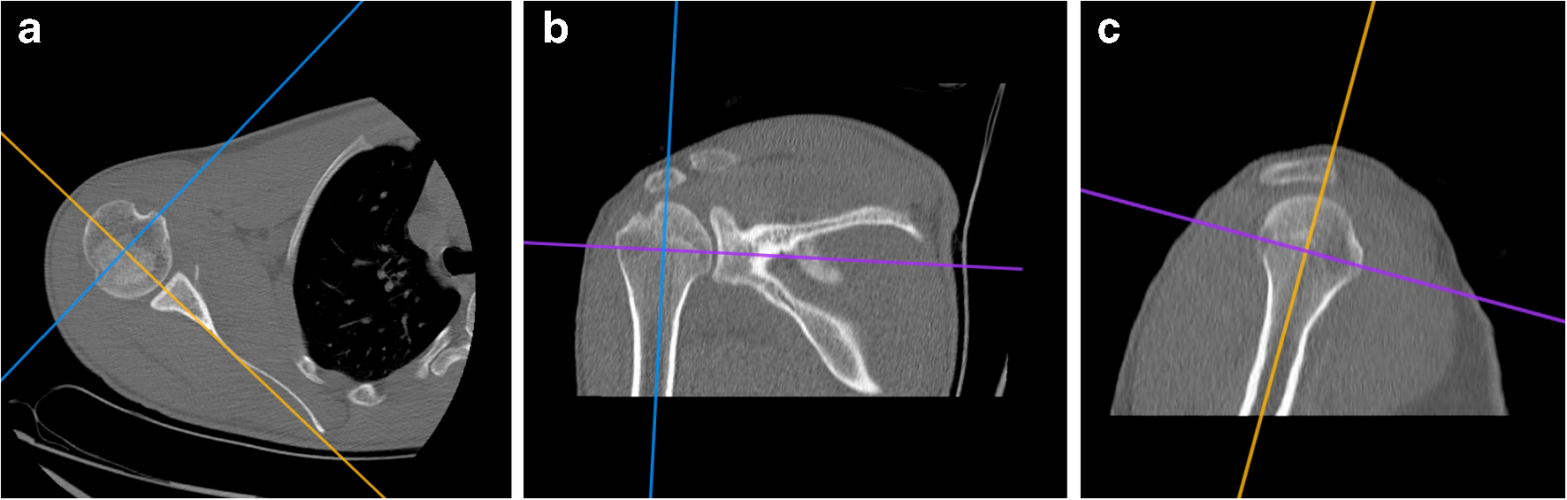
Initial 2D-CT multiplanar reconstruction for humeral head measurements. The sagittal (c) and frontal plane (b) axes run along the long axis of the humerus; the third axis on the transverse plane (a) marks the long axis of the humeral head (Osirix MD)
